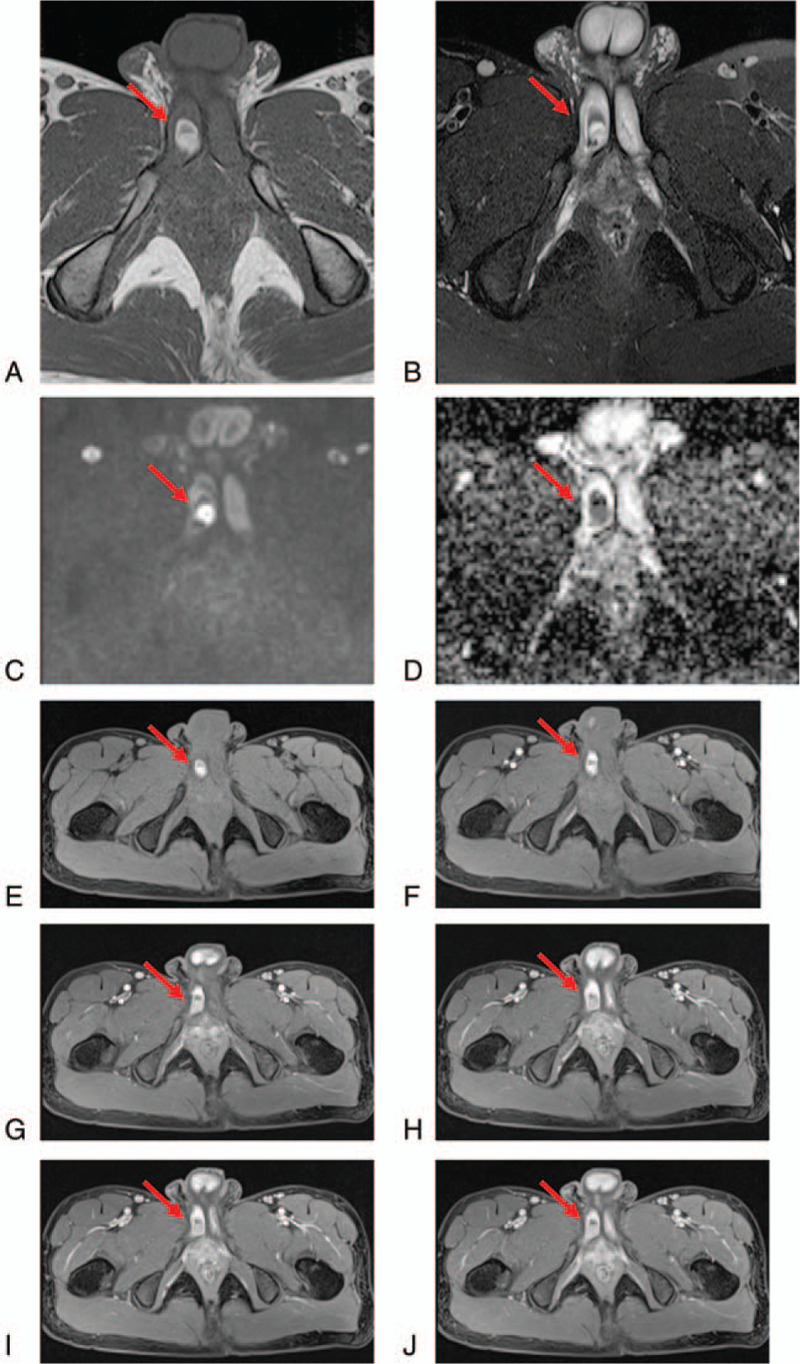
Nodular abnormal signal shadows at the right corpus cavernosum root. A, T1WI dominated by high signals, accompanied by internal low-signal shadows. B, FS-T2WI showing uneven circular nodular high signals, surrounded by low-signal shadows. C, Diffusion-weighted imaging (DWI) showing obvious high signals. D, Apparent diffusion coefficient (ADC) suggesting low signals. E–J, Contrast-enhanced scanning showing progressive and significant enhancement inside and around the above abnormal signals, with the right corpus cavernosum displayed with continuous enhancement.
